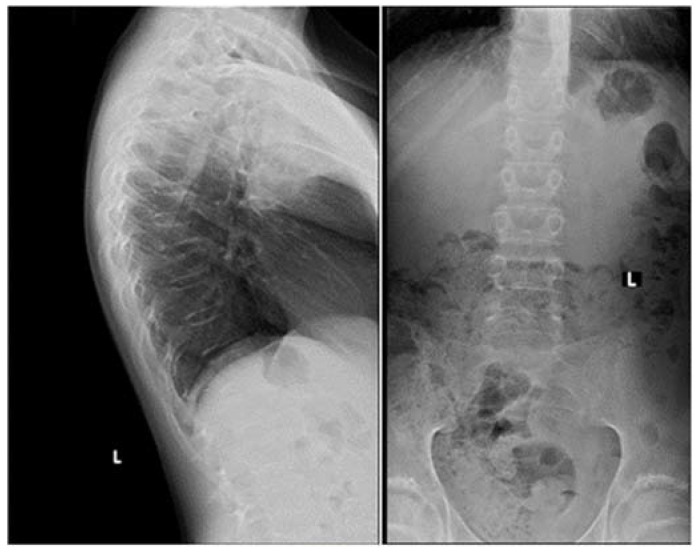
A thoracolumbar spine X-ray with multilevel vertebral fractures. Decrease in the height of the Th5–Th9 vertebrae and central lowering of the upper border plate of the L4 and L5 were found.
